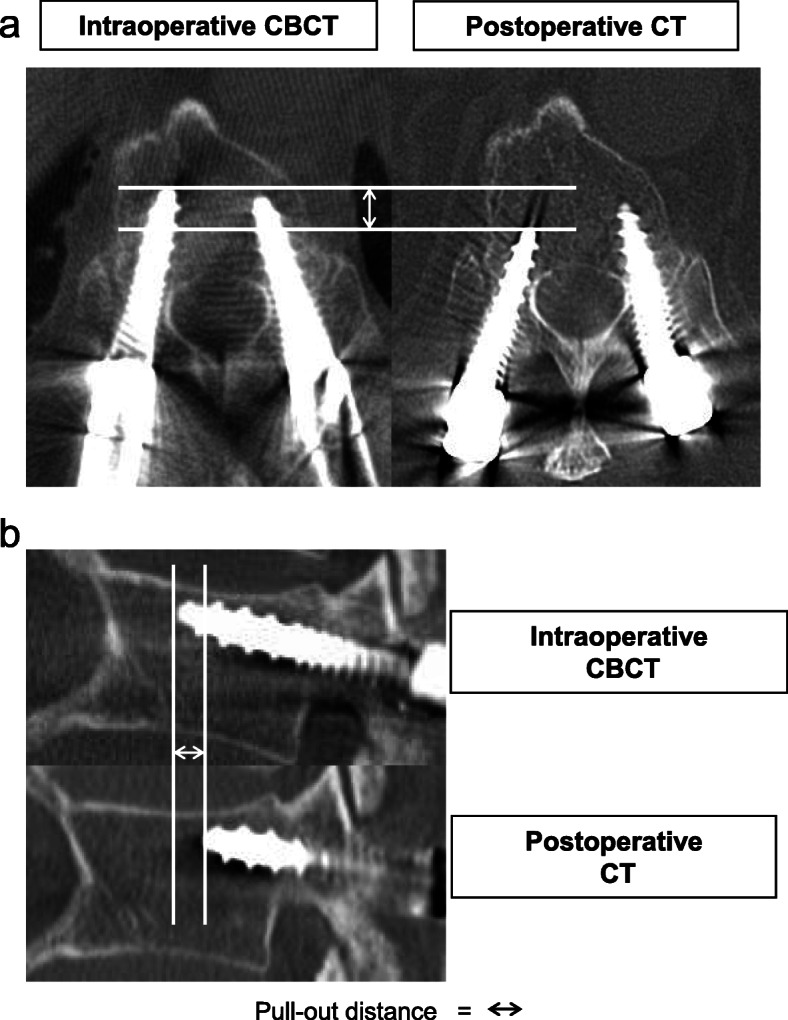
Evaluation of pedicle screw pullout distance. Pedicle screw pull-out was defined as a distance of > 2 mm in the (a) axial and (b) sagittal CBCT and CT planes. CBCT, cone-beam computed tomography; CT, computed tomography
